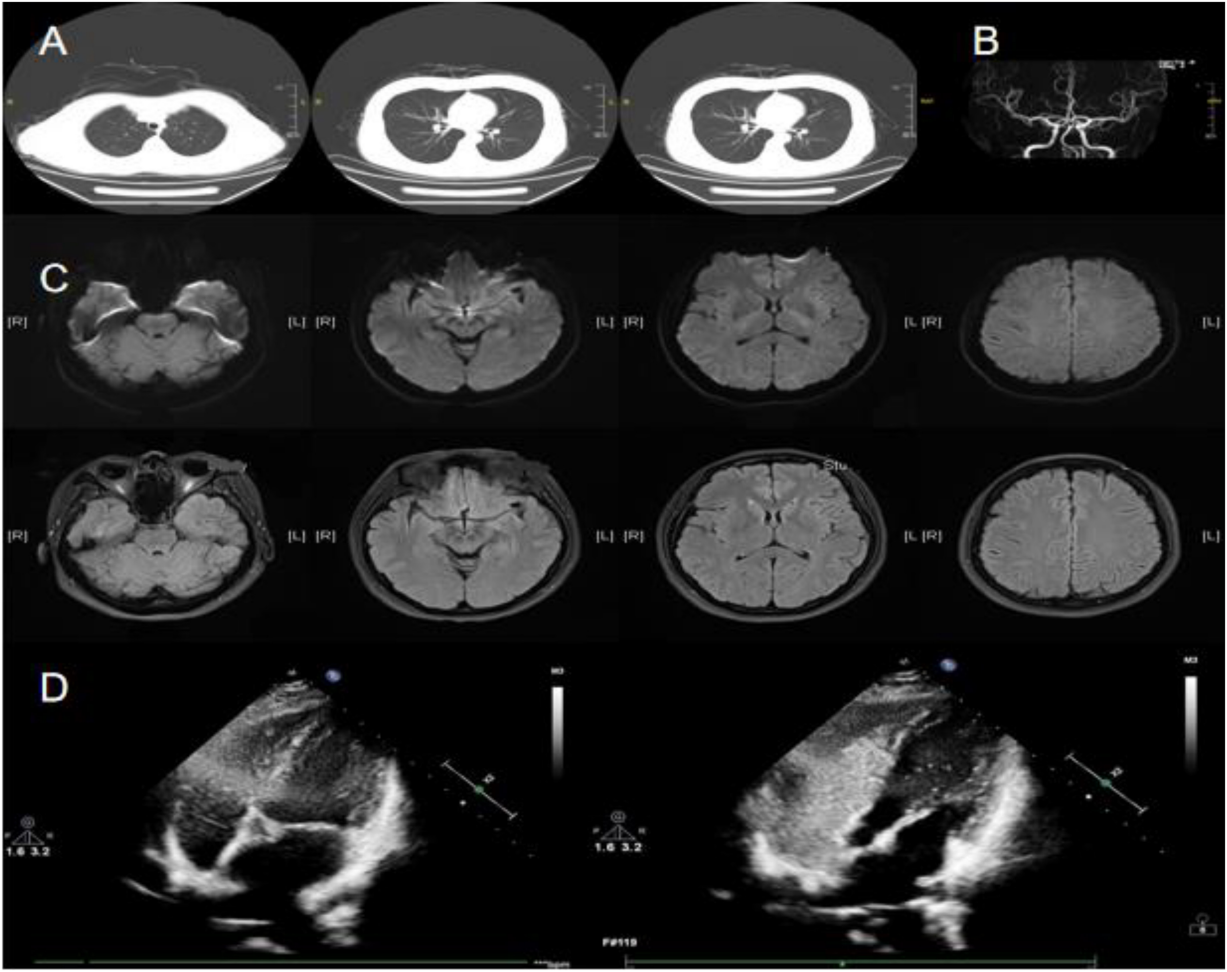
Main examination findings for case 1: (A) chest CT; (B) cranial MRA; (C) cranial MRI; (D) right heart catheterization imaging.
